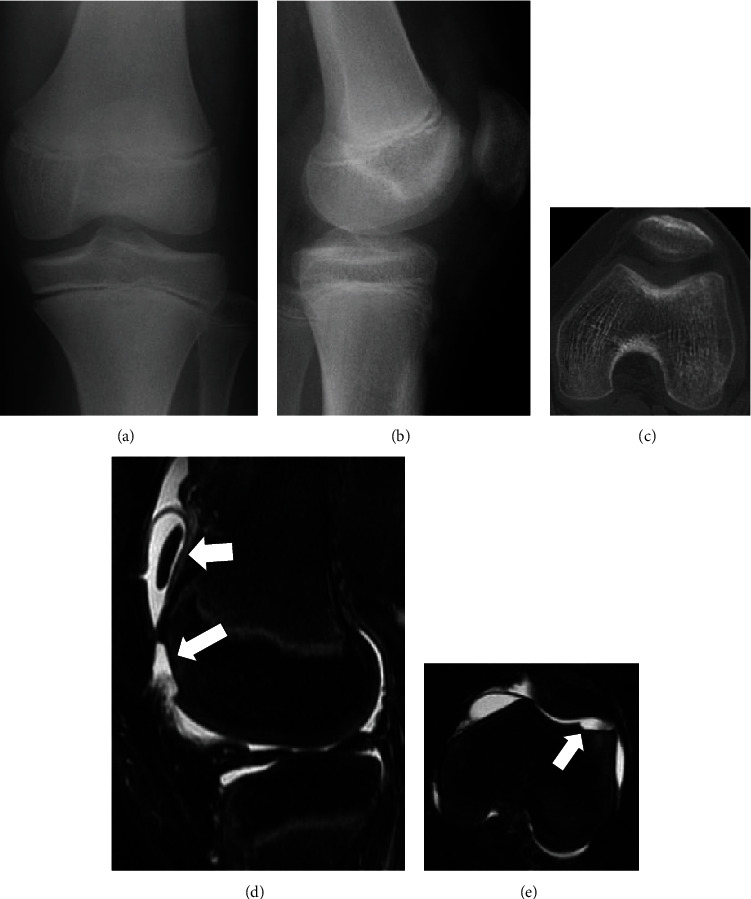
Representative images of case 2. Preoperative anteroposterior (a) and lateral (b) radiography and axial computerized tomography (c) of the knee showing no bony injury. Preoperative sagittal (d) and axial (e) T2-weighted, fat-suppressed magnetic resonance imaging showing a chondral defect in the lateral femoral condyle and a loose chondral fragment in the medial suprapatellar pouch.
